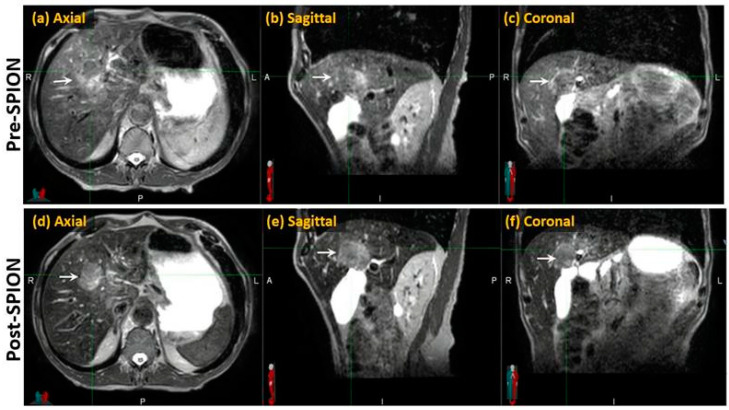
Pre- and post-SPION MR images of HCC (arrows) in the first and second row, respectively.
